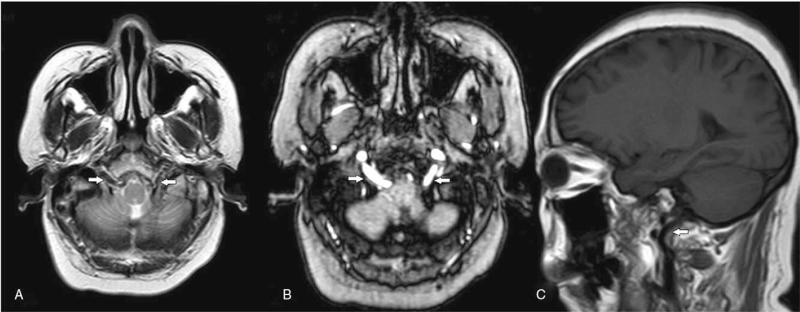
Brain MRI showing bilateral dilated hypoglossal canals, with PHAs (white arrows) traversing these (A, B). Right PHA (white arrow) originating from ICA and entering the skull through hypoglossal canal (C). MRI = magnetic resonance imaging.
